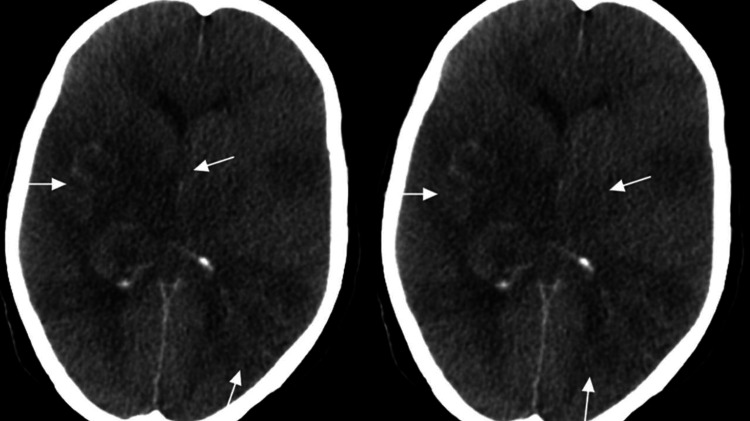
Brain computed tomography (CT) CT brain revealed significant interval worsening of the vasogenic edema with interval development of cytotoxic edema causing a mass effect with cerebellar tonsillar herniation, bilateral uncal herniation, and possible venous sinus thrombosis.
